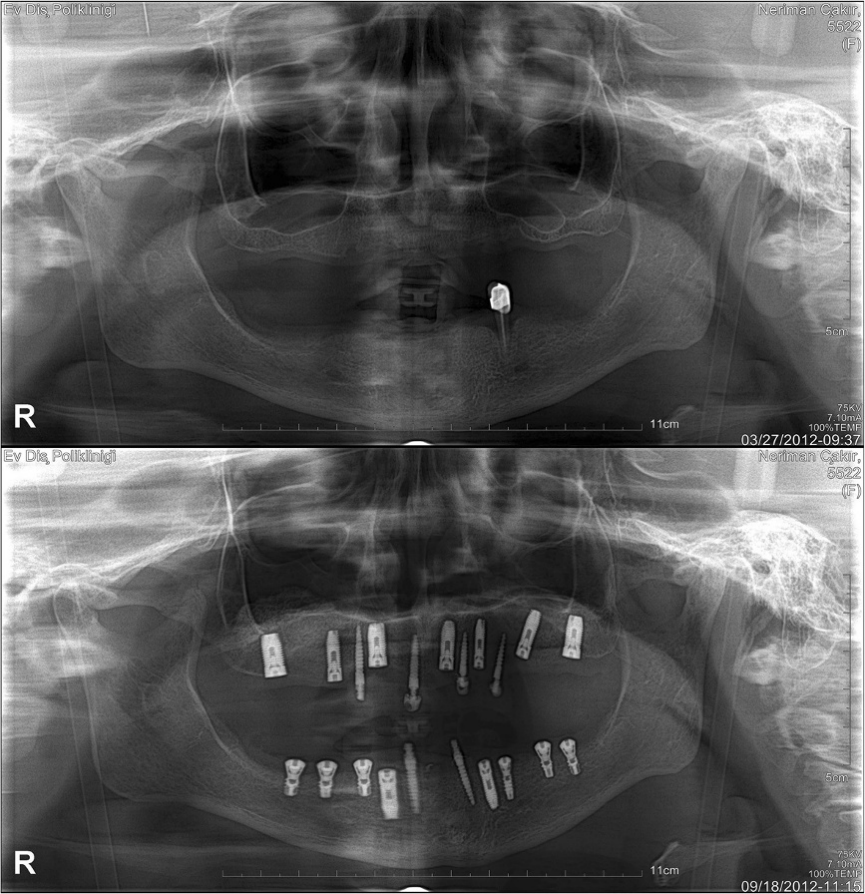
An edentulous patient’s comparative panoramic x-rays before and after dental implant treatment.
